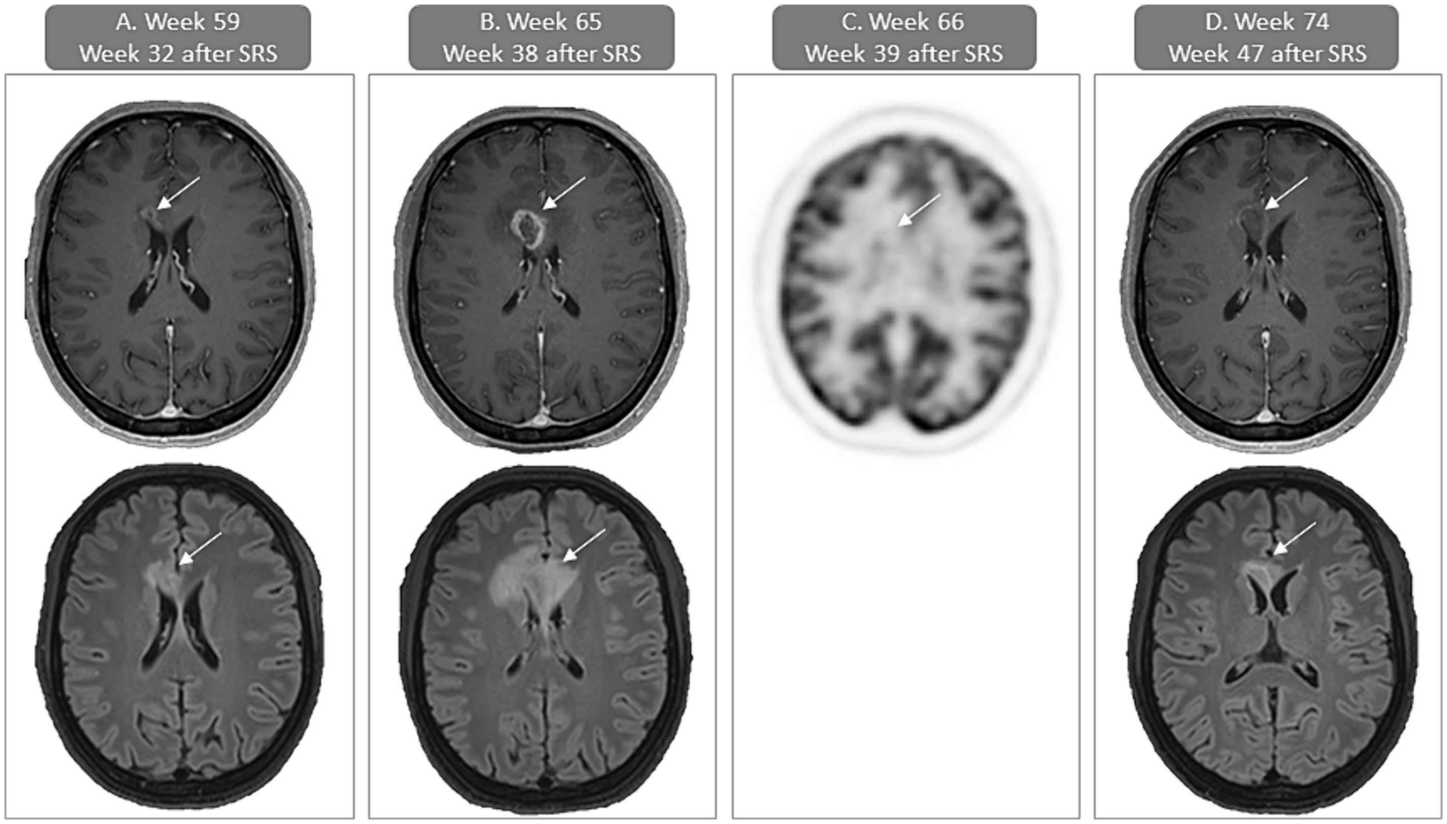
Case presentation of focal post-radiation necrosis of the brain (fRNB) with gadolinium contrast-enhanced T1-weighted and FLAIR MRI of the brain and [^18^F]FDG-PET/CT (FDG-uptake images). (A) First sign of fRNB (week 59 or 32 weeks after SRS) with contrast-enhancement in the paramedian frontal right region on the T1-weighted axial image (upper) and surrounding edema on the FLAIR axial image (lower). (B) In week 65 or 38 weeks after SRS there is an increase of contrast-enhancement in the paramedian frontal right region on the T1-weighted axial image (upper) and surrounding edema on the FLAIR axial image (lower). (C) PET-CT performed 1 week later (week 66 or 39 weeks after SRS) shows no increased uptake of [18F]FDG, supporting the diagnosis of fRNB. (D) In week 74 or 8 weeks after bevacizumab initiation there is a decrease of contrast-enhancement in the paramedian frontal right region on the T1-weighted axial image (upper) and surrounding edema on the FLAIR axial image (lower). SRS, stereotactic radiosurgery.
